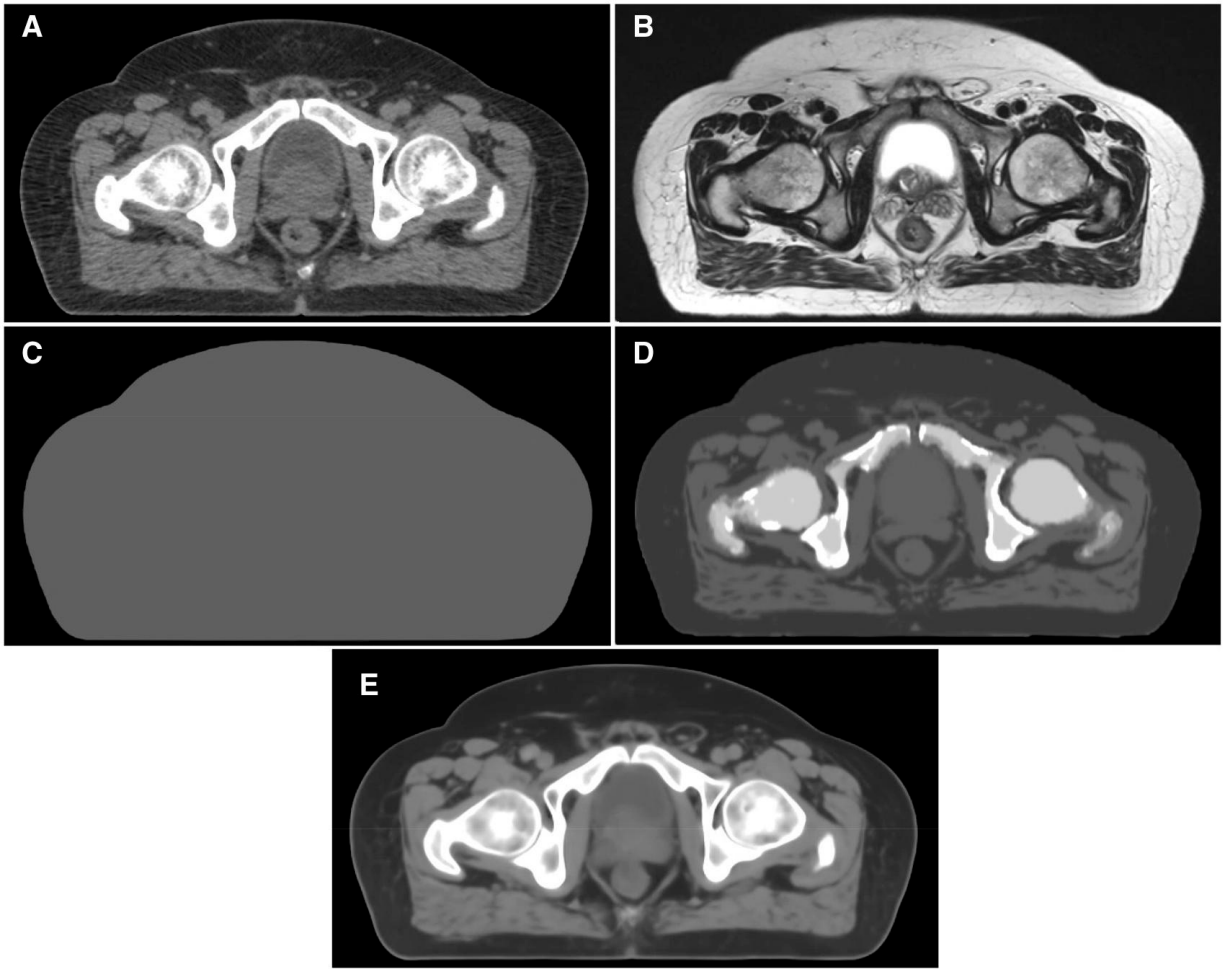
Axial slice from representative patient showing (A) planning CT, (B) T2tse, (C) sCT_BDw, (D) sCT_TS, and (E) sCT_AI.
